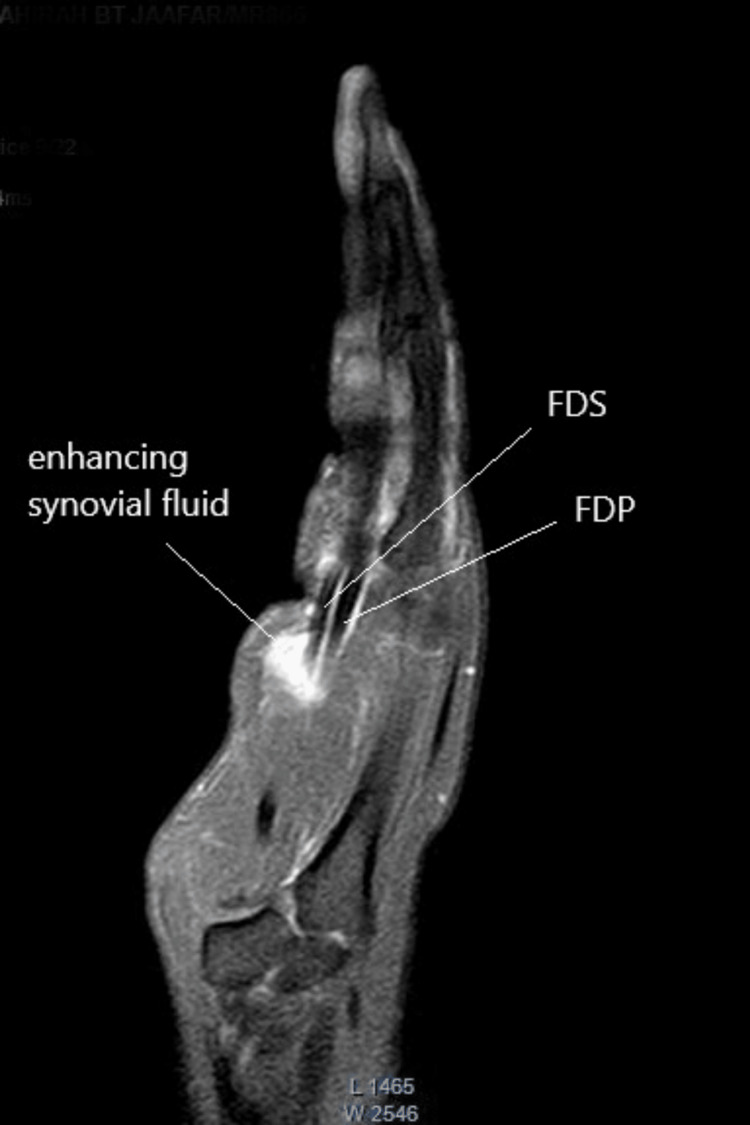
MRI sagittal view of the left hand. Note the flexor tendons remained in integrity. The enhancing signal appeared along the synovial sheath. FDS: flexor digitorum superficialis; FDP: flexor digitorum profundus; MRI: magnetic resonance imaging
